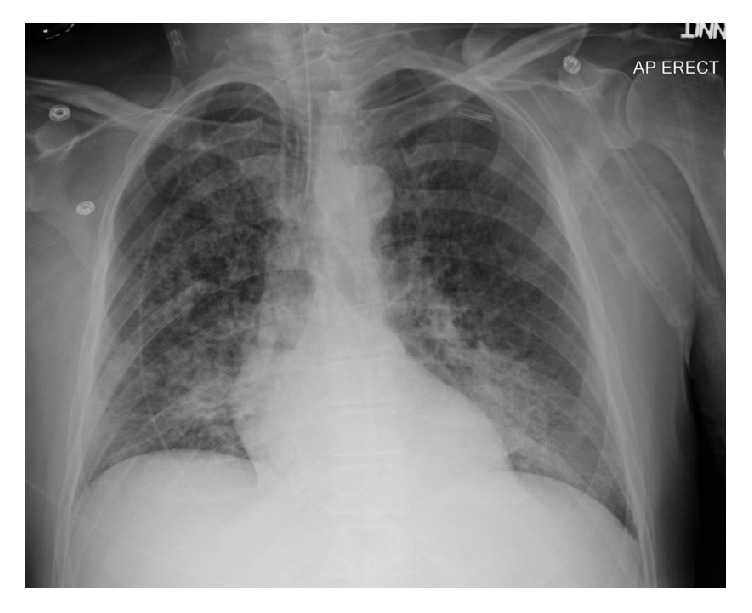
Chest X-ray showing features of pulmonary edema.
